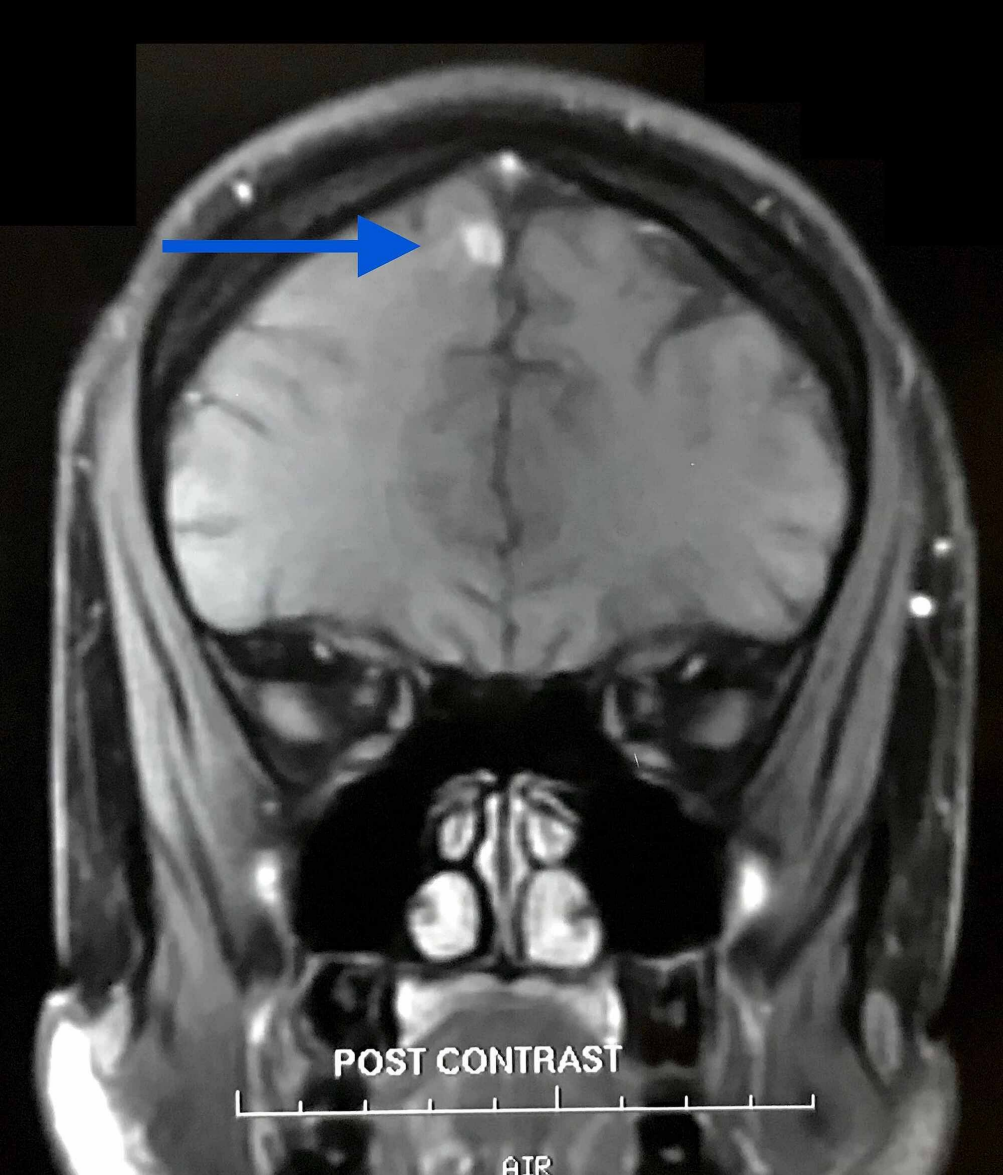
Neurocysticercosis in the right frontal lobe of the brain, post-contrast coronal MRI.
